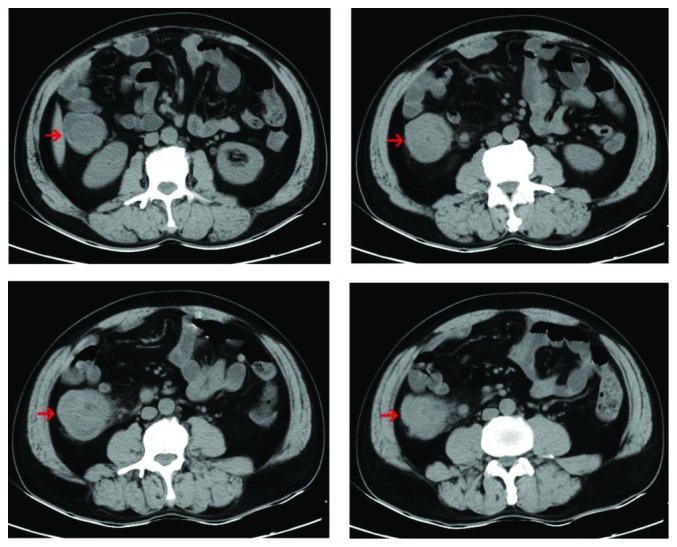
Abdominal CT of Case 9 showing an ascending colon mass and surrounding lymph node enlargement, which was suggestive of the possibility of colon cancer.
